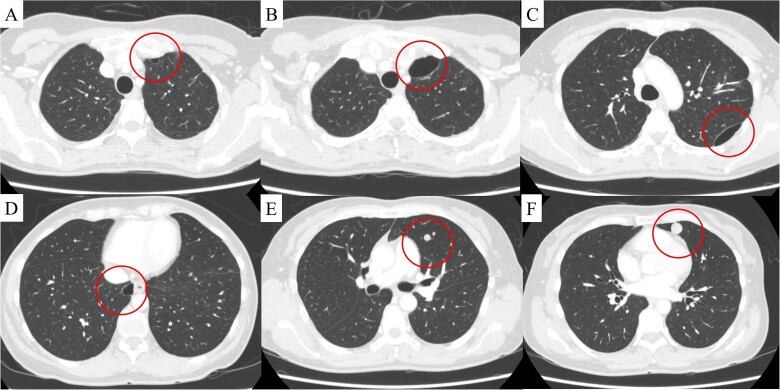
(A) Computed tomography revealed a bulla in the left upper lobe 1.5 years after uterine surgery. At 3 years, in addition to the development of the bulla in the left upper lobe (B), two more bullae appeared and gradually developed in the left (C) and right lower (D) lobes, and two pulmonary metastases appeared in the left lingular segment (E, F). The findings are indicated by circles.
